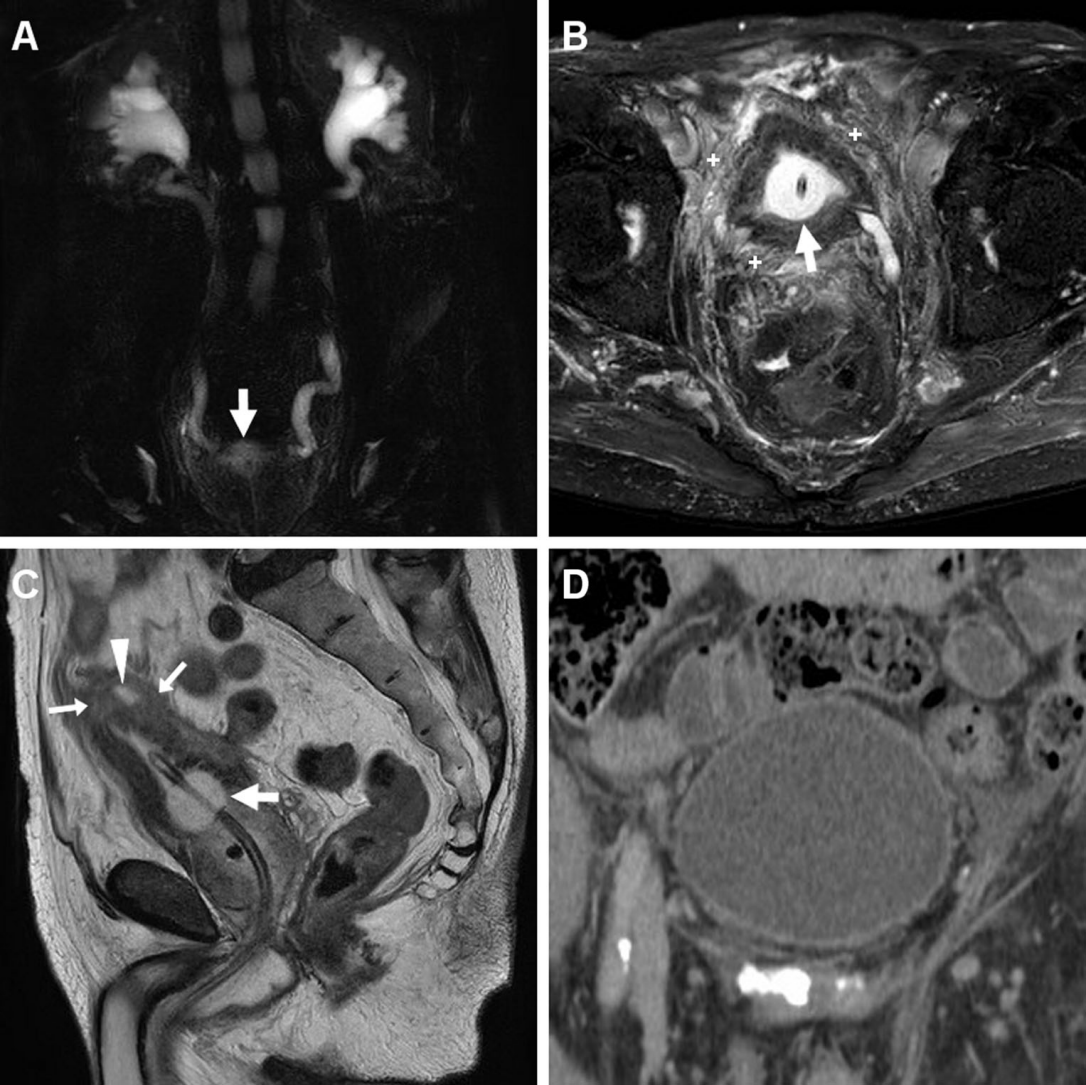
Acute infectious cystitis with mural bladder abscess in an 89-year-old man with acute urinary retention, fever, leukocytosis and impaired renal function. Unenhanced MRI including MR pyelographic (a) and axial fat-suppressed (b) images revealed bilateral hydronephrosis, contracted bladder and prominent inflammatory changes (+) in the surrounding extraperitoneal fat planes. Additionally, sagittal T2-weighted image (c) showed a focal thickening (*arrows*) at the bladder dome, with intramural fluid collection (*arrowhead*). Repeated CT (d) after medical treatment revealed the disappearance of abnormal mural changes
